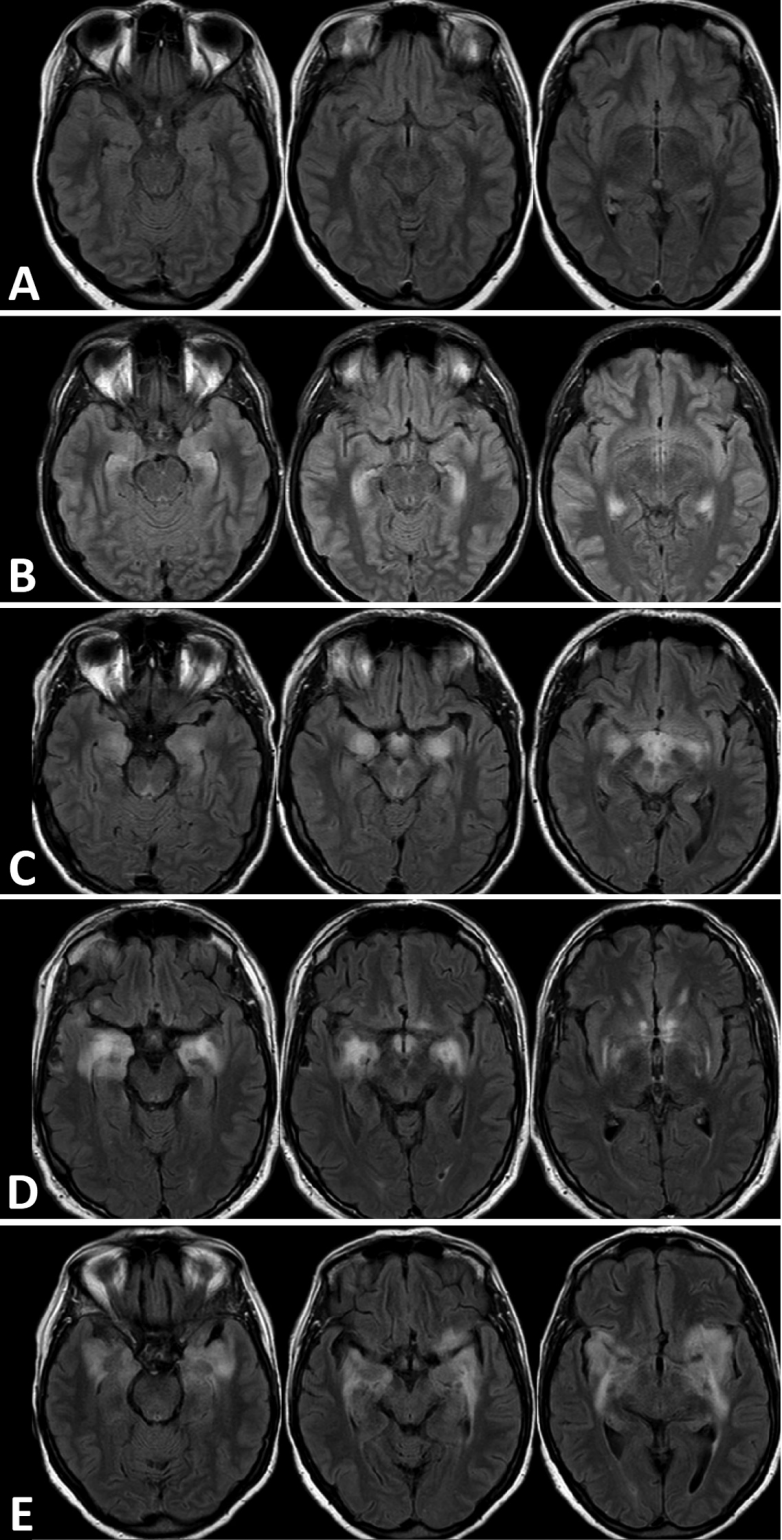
T2-weighted-fluid-attenuated inversion recovery (T2-FLAIR) brain magnetic resonance imaging (MRI) scans. (
